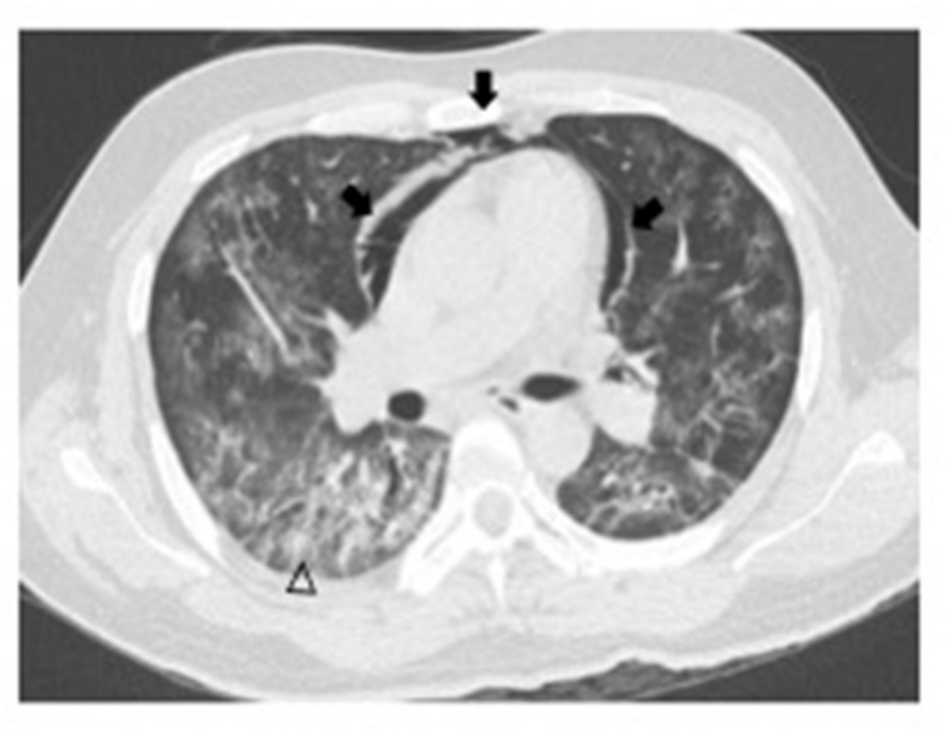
A 43-year-old male non-enhanced chest CT image showing pneumomediastinum (arrows) and ground glass opacity pattern over peripheral subpleural areas (arrowhead).
